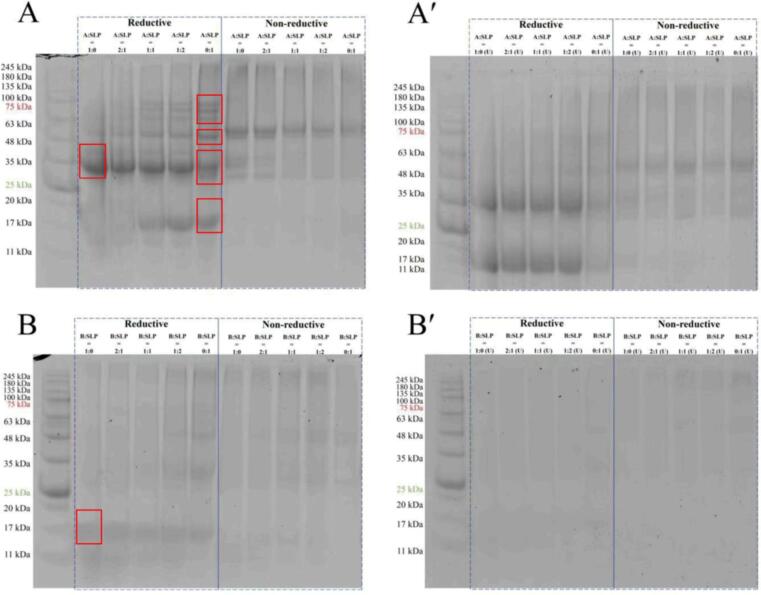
Non-reductive and reductive SDS-PAGE profiles of soy protein gels before and after ultrasound.(Figure A shows the reductive and non-reductive electrophoresis of different proportions of A-SLP before sonication and Figure A' shows the reductive and non-reductive electrophoresis of different proportions of A-SLP after sonication; Figure B shows the reductive and non-reductive electrophoresis of different proportions of B-SLP before sonication and Figure B' shows the reductive and non-reductive electrophoresis of different proportions of B-SLP after sonication).
